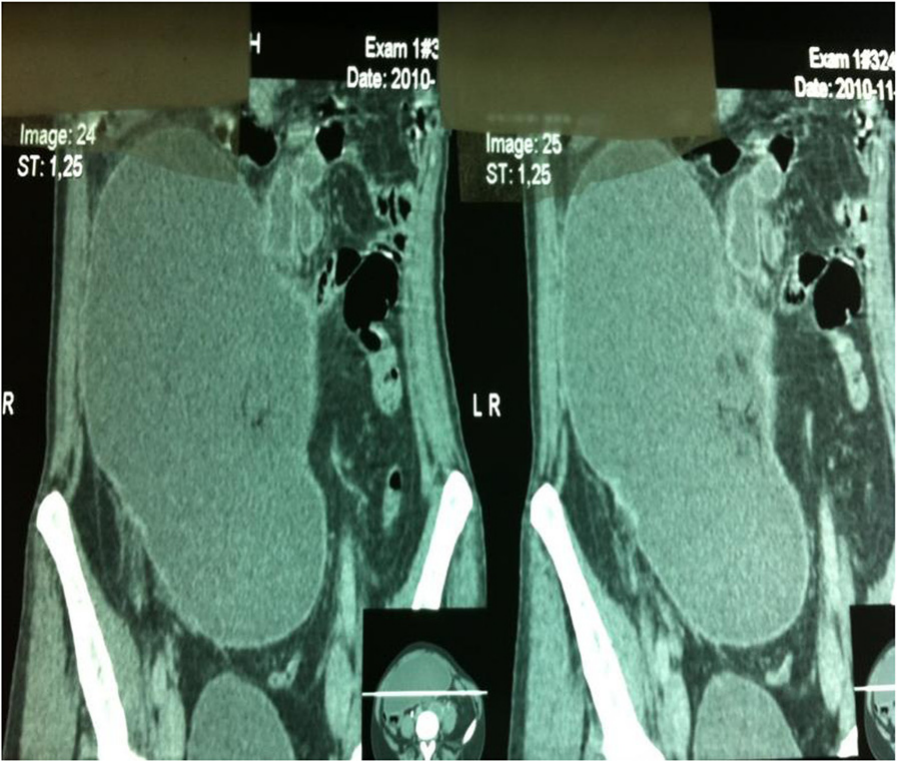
Anteroposterior Angio-CT showing enlarged spleen in lower right hemiabdomen.
